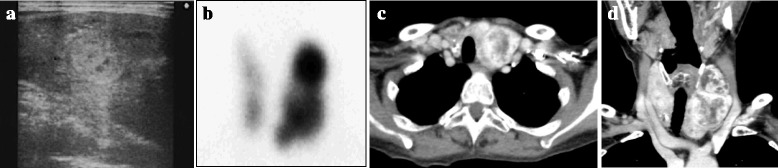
Imaging findings of the thyroid gland. Ultrasonography demonstrated multiple nodules in the thyroid gland (a). The thyroid nodules showed an increased uptake of ^99m^Tc on thyroid scintigraphy (b). The axial (c) and coronal view (d) of the thyroid gland on contrast-enhanced computed tomography showed heterogeneously enhanced multiple tumors with microcalcifications
